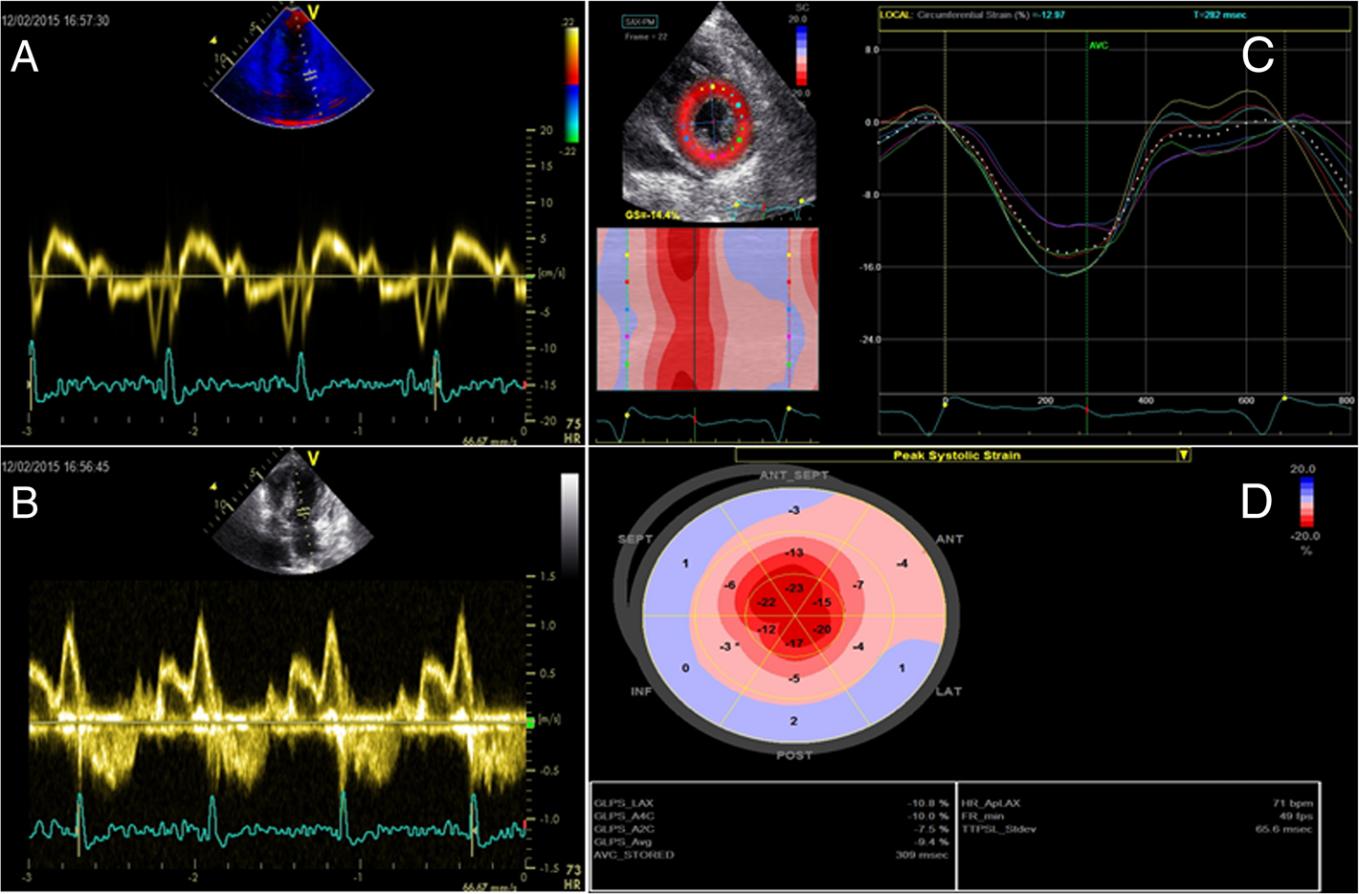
Mitral valve inflow pulsed wave Doppler (a) and Tissue Doppler Imaging of the mitral valve annulus (b) of a patient with CA demonstrating an early diastolic dysfunction pattern. Circumferential (c) and longitudinal deformation by 2D strain imaging (c and d) mainly shows an impairment of global longitudinal deformation. In patients with CA, an impaired deformation in basal segments compared to apical segments could be found
